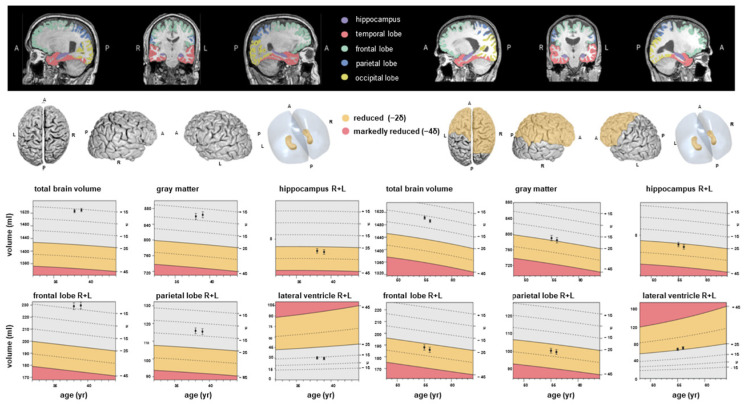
Examples of fully automated, artificial intelligence (AI)-based brain volumetry in two patients with severe course of COVID-19 (left: patient 1, right: patient 2) in time sequelae: baseline and follow-up MRI. Various brain volumes are displayed together with their deviations from age- and sex-adjusted normative values. The colour scale indicates the degree of volume reduction, with yellow representing moderate deviation (~2 SD) and red indicating marked deviation (~4 SD). A, anterior, P, posterior, L, left, R, right.
